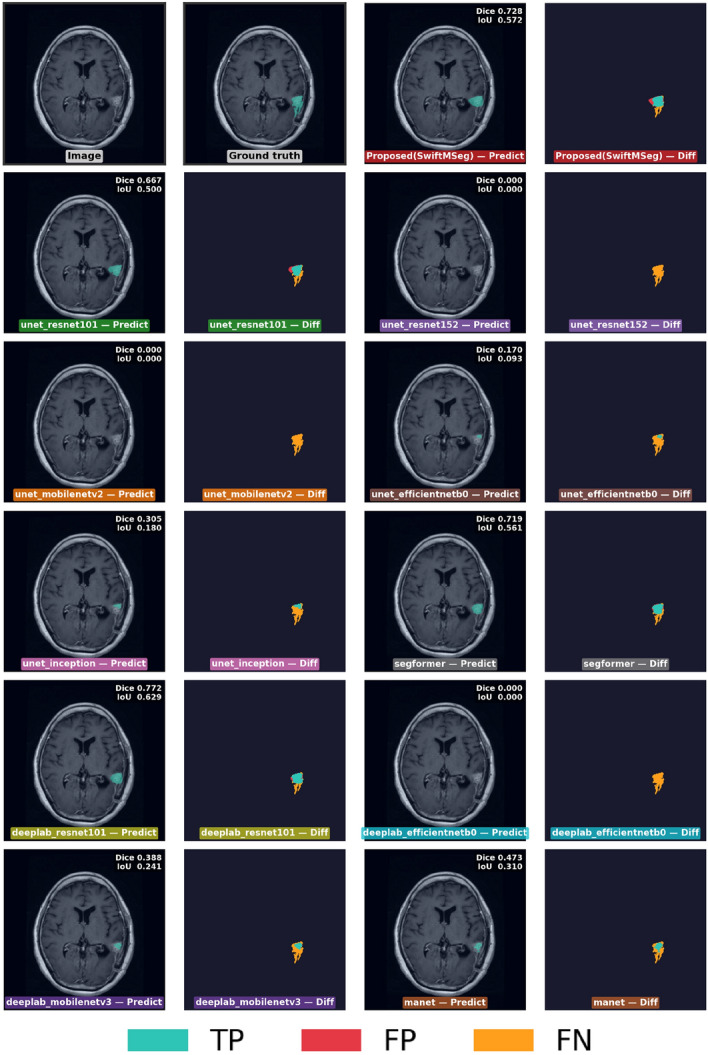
Brain tumor segmentation in MRI image (BRISC^49^) using different models (*TP* true positive, *FP* false positive and *FN* false negative).
